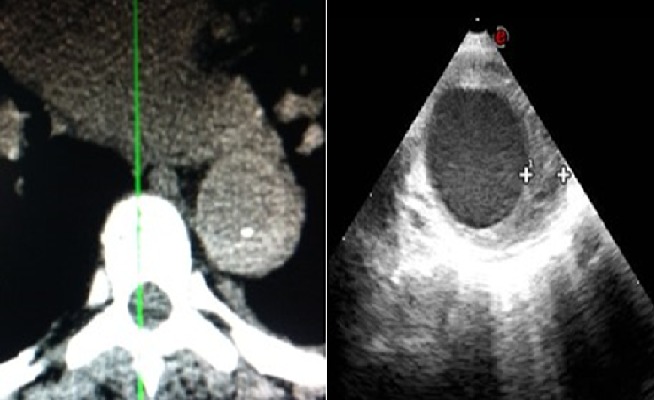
Type B hematoma formation in the descending thoracic aorta with internal deviation of intimal calcification. At left: non-contrast computed tomography. At right: TEE showing tickening of aorta
